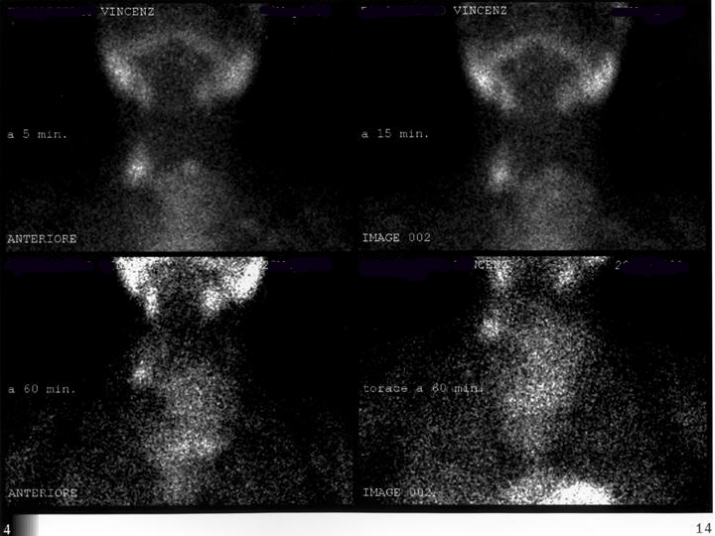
Sestamibi scintigraphy: diffuse enlargement of the thyroid gland associated with irregular uptake of the radionuclide.
